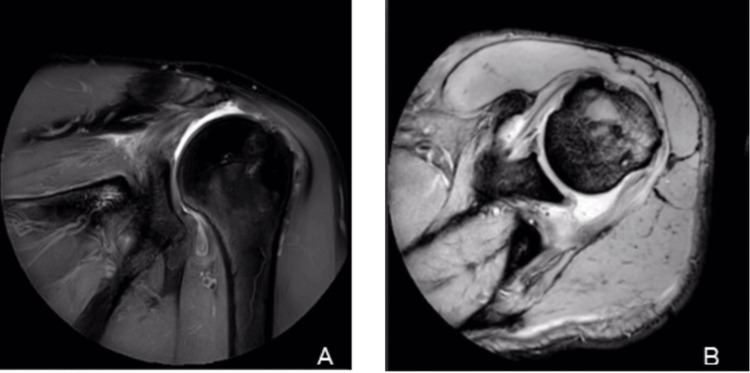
T2-weighted magnetic resonance imaging of the left shoulder (A) Sagittal view showing a retear of the posterior supraspinatus at the myotendinous junction with tendon retraction. (B) Axial view demonstrating extension of the tear to the infraspinatus
